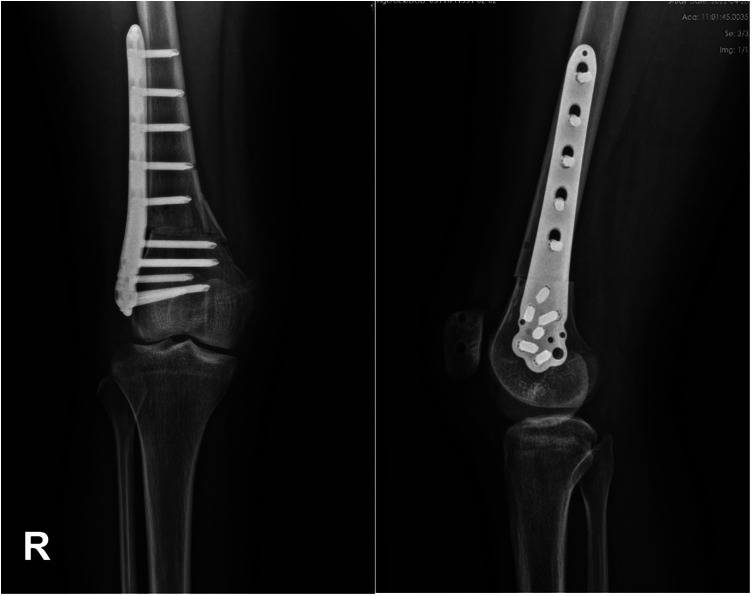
Radiograph and CT scan after MPFLR combined with DDFO. MPFLR, medial patellofemoral ligament reconstruction; DDFO, derotational distal femur osteotomy.
